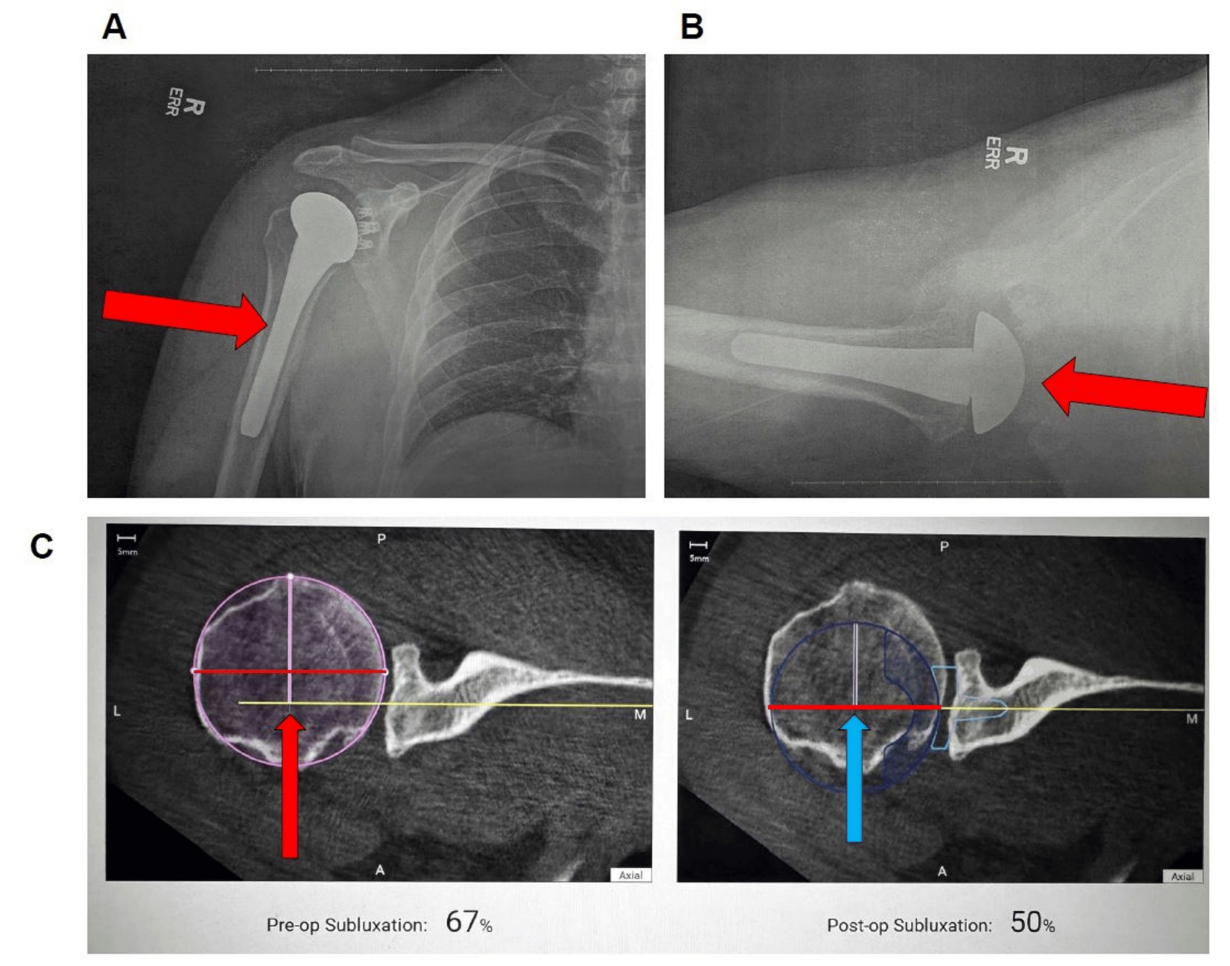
Postoperative imaging following an anatomic total shoulder arthroplasty performed using the ExactechGPS navigation system (Exactech, Inc., Gainesville, FL). (A) Anteroposterior radiograph demonstrating anatomic restoration of joint alignment and appropriate humeral head prosthesis positioning. (B) Axillary lateral view confirming concentric reduction and neutral version of the glenoid component without evidence of loosening or malposition. (C) Axial CT comparison demonstrating correction of posterior humeral head subluxation from 67% preoperatively (red arrow) to 50% postoperatively (blue arrow) following optimized glenoid version and implant placement.
